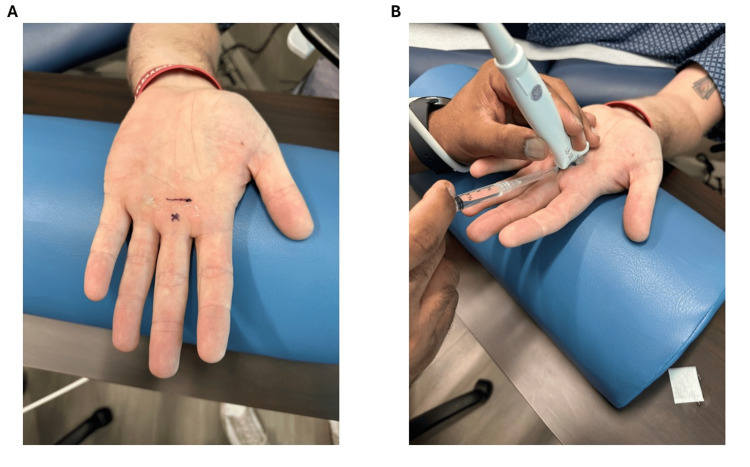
(A) Surface markings identifying the needle insertion point (X) and the level of the A1 pulley (line). (B) Ultrasound-guided needle placement for percutaneous A1 pulley fenestration
